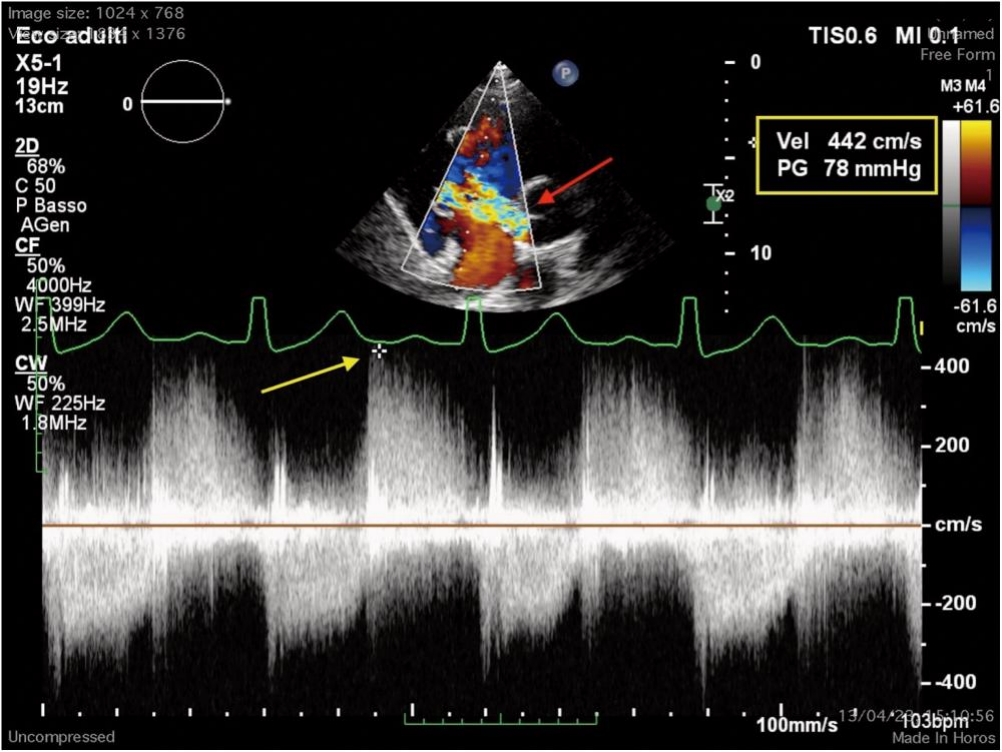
TTE (short axis), colour to study fistula and left–right shunt (red arrow), Doppler to study shunt’s maximum velocity and gradient (yellow box and yellow arrow).
